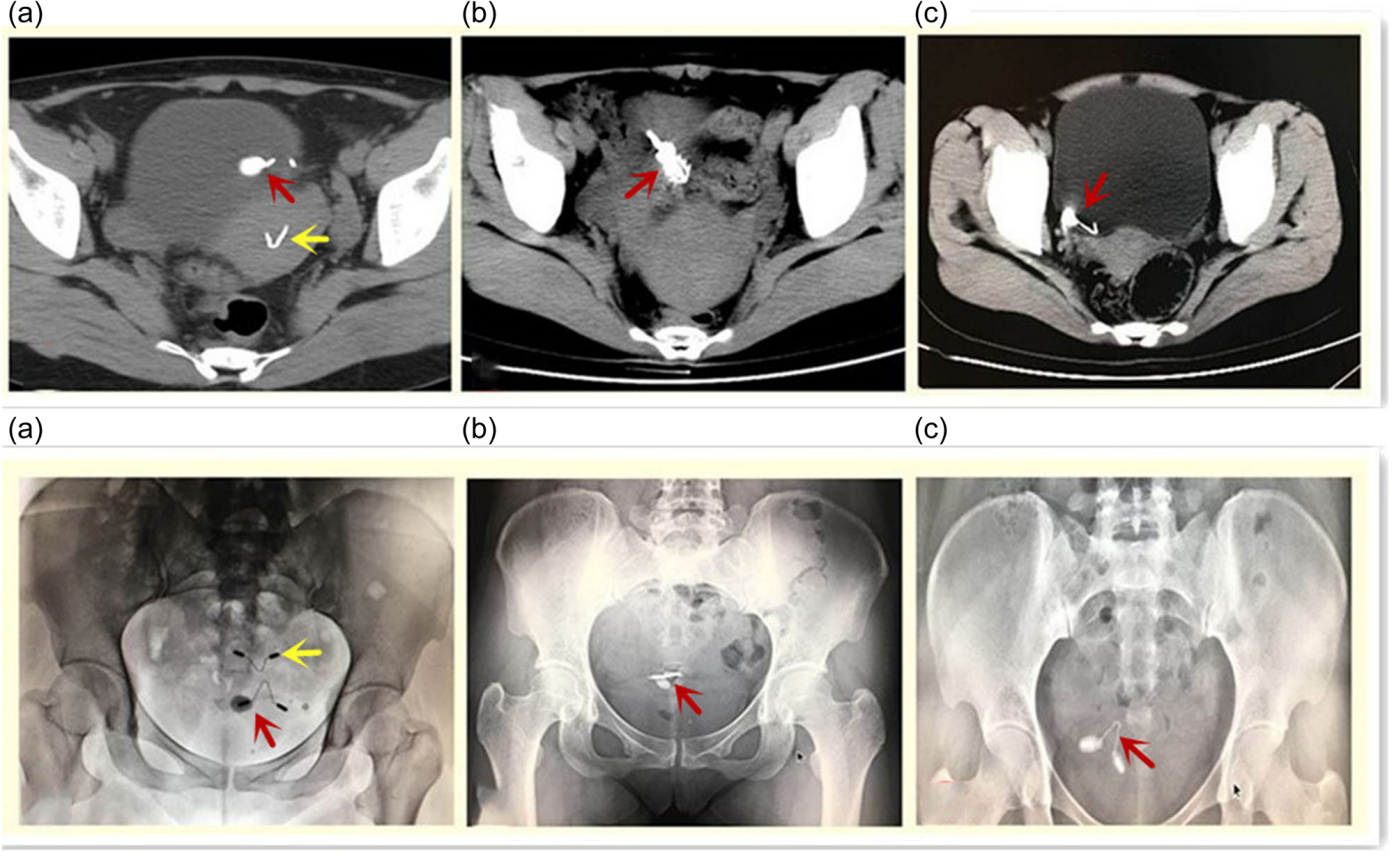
The ectopic IUD was examined by pelvic radiography and computed tomography (CT) and was found to be embedded in the bladder wall and wrapped with a calculus. (a) A 34-year-old patient, gravida 2, para 2 (case #1). The patient got pregnant after IUD insertion. After the induced abortion, a new IUD was inserted. The patient suffered from abdominal pain and hematuria for half a year. Two IUDs can be seen, one is in the uterus (yellow arrow), and the other is embedded in the left posterior wall of the bladder (red arrow). (b) A 33-year-old patient, gravida 2, para 1 (case #2). The patient suffered from abdominal pain and hematuria for 1 year. The ectopic IUD was completely embedded in the posterior wall of the bladder (red arrow). (c) A 34-year-old patient, gravida 2, para 2 (case #5). The patient suffered from intermittent hematuria and urodynia for 9 months. The IUD was completely shifted in the bladder (red arrow).
